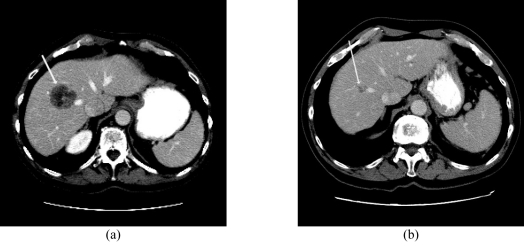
Partial response to Y90 microsphere therapy with the use of 1.61 GBq glass microspheres in an 83 yr old man with metastatic colorectal cancer; Contrast enhanced CT scan (a) before treatment demonstrates a low attenuation area in the right lobe of the liver (arrow) consistent with a metastasis with (b) significant reduction in size 12 months after treatment.
